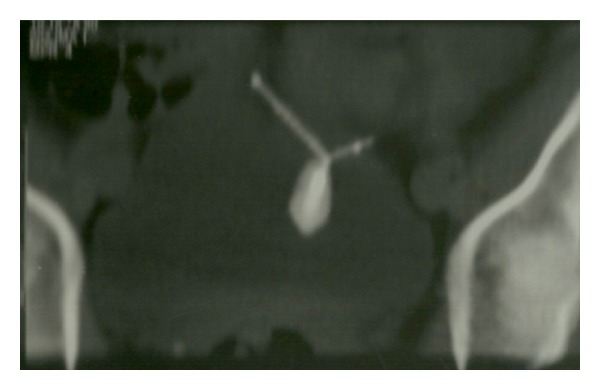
Pelvic helical computed tomography revealed a metallic foreign body partially located intravesically with adjacent calcification.
